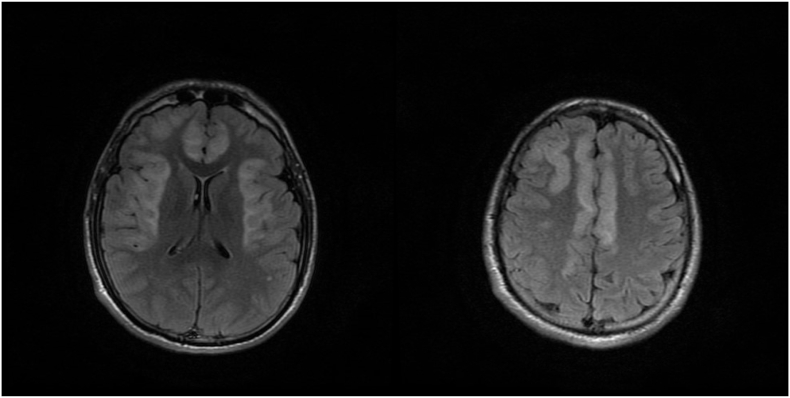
A and B: Axial T2 FLAIR MRI showing hyper intensities in the frontal and temporal lobes.
